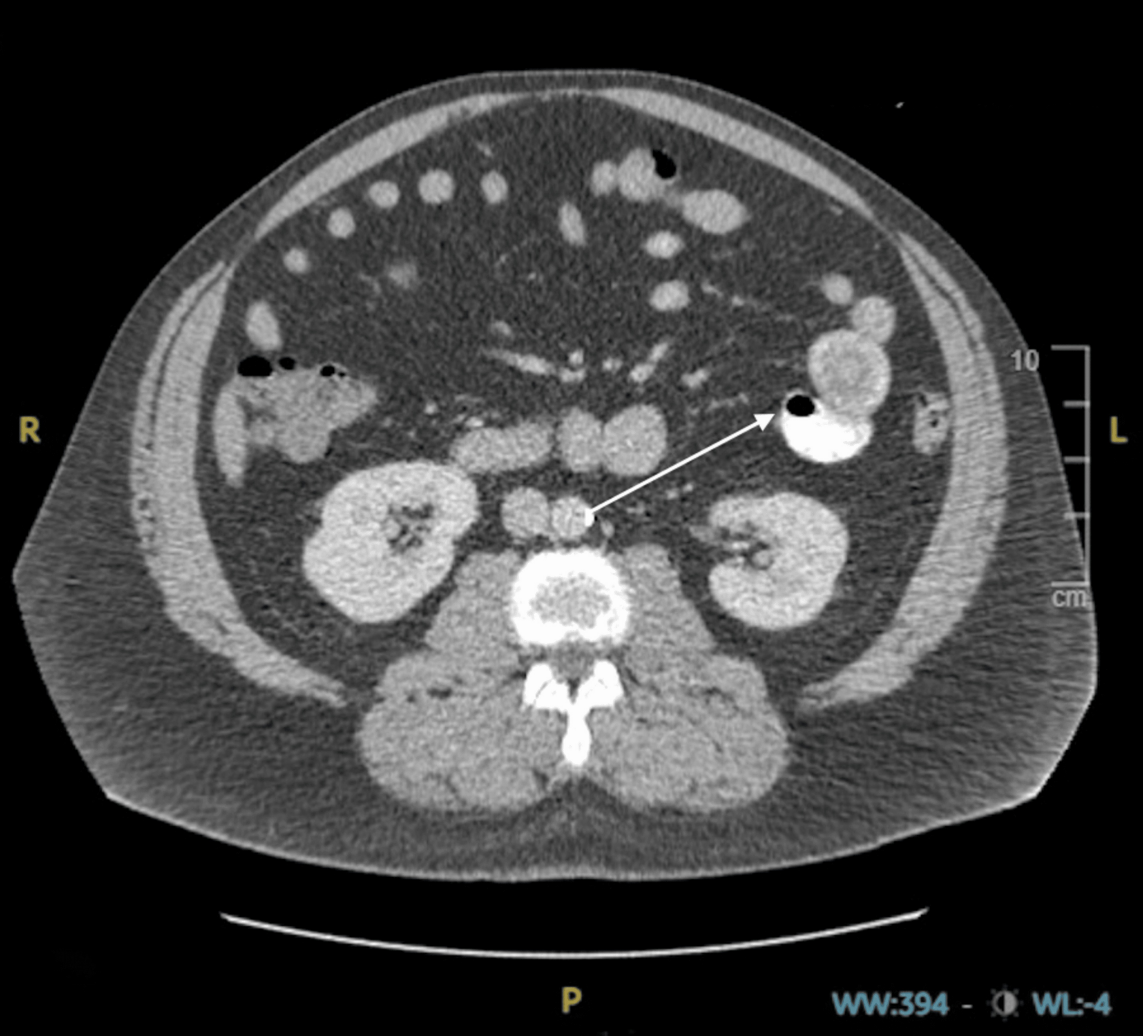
CT scan of the abdomen demonstrating small bowel to small bowel intussusception CT: computed tomography
